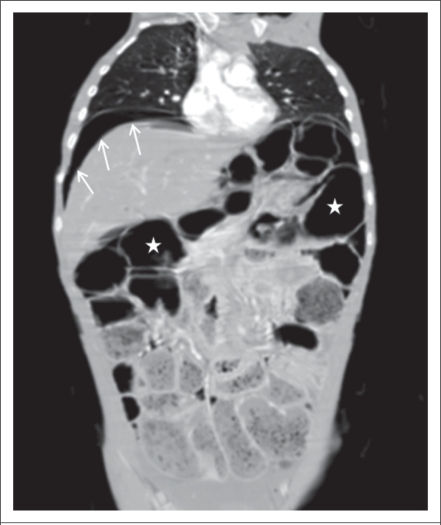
Coronal CT image showing extensive small-bowel pneumatosis (stars), pneumoperitoneum (white arrows), and axial CT image
